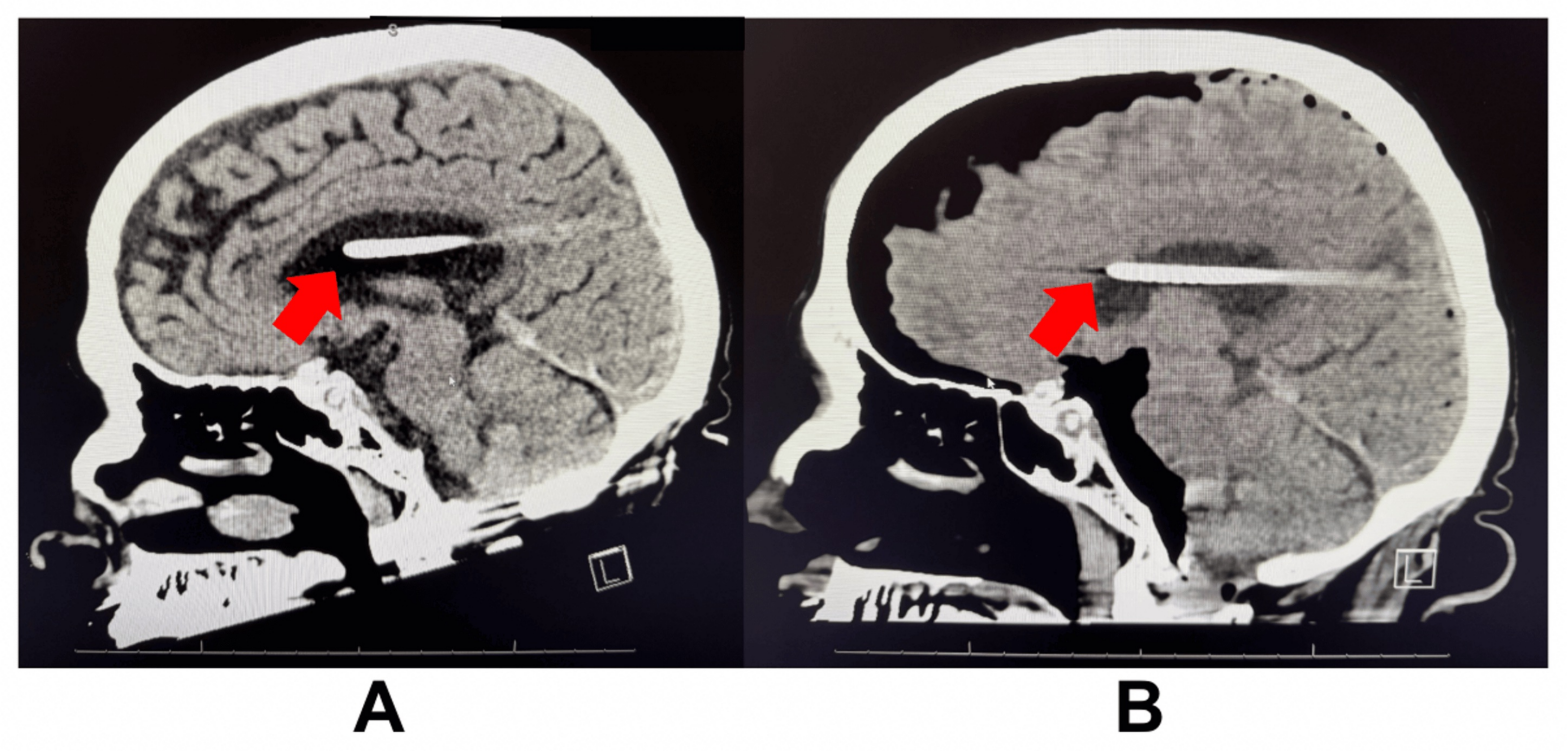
Non-contrast CT brain depicting the shift in VP shunt. Picture A shows the VP shunt inside the lateral ventricle from imaging during a prior admission. Picture B shows the VP shunt shifted slightly anterior compared to the position in picture A. The anterior tip of the VP shunts is indicated by the arrows.
